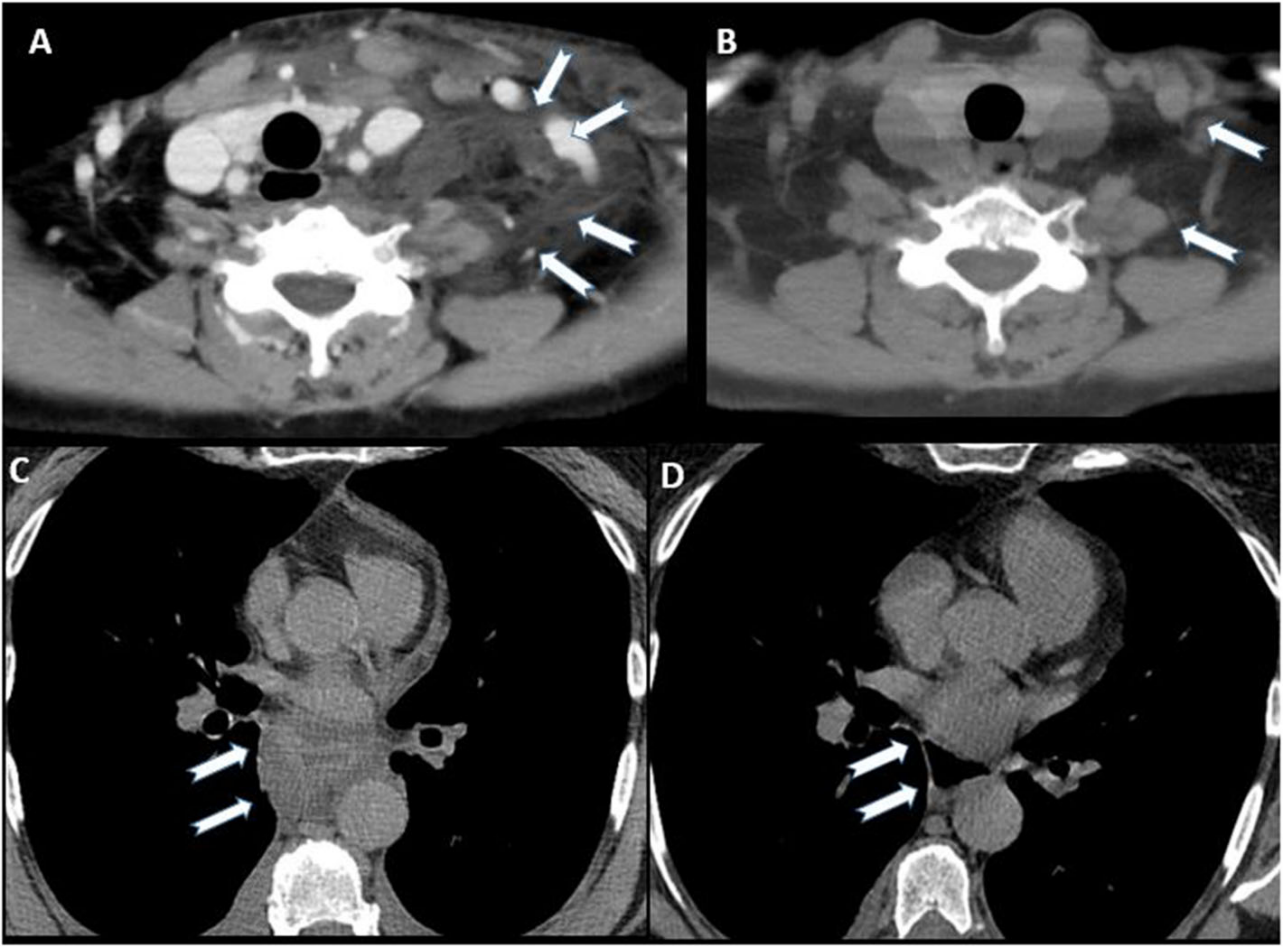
Computed tomography images. Axial contrast-enhanced and non-contrast neck CT images on the admission and discharge (A,B) showed soft-tissue swelling and diffuse reticulation of subcutaneous fat with edema around vessels (arrows in A) with a complete resolution in few days (arrows in B). Axial contrast-enhanced and non-contrast chest Computed Tomography images on the admission and discharge (C,D) documented esophageal wall edema with adjacent effusion (arrows in C) with a complete resolution in few days (arrows in D)
